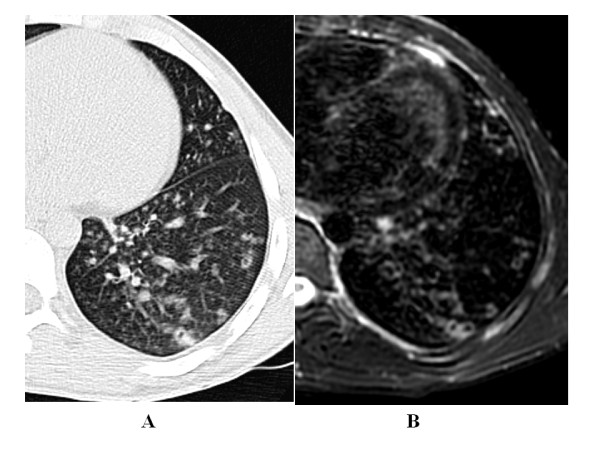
44 year-old man with pulmonary tuberculosis. A) HRCT shows interstitial changes with peribronchial thickening. B) Unenhanced MRI identifies the same features as well as HRCT.
